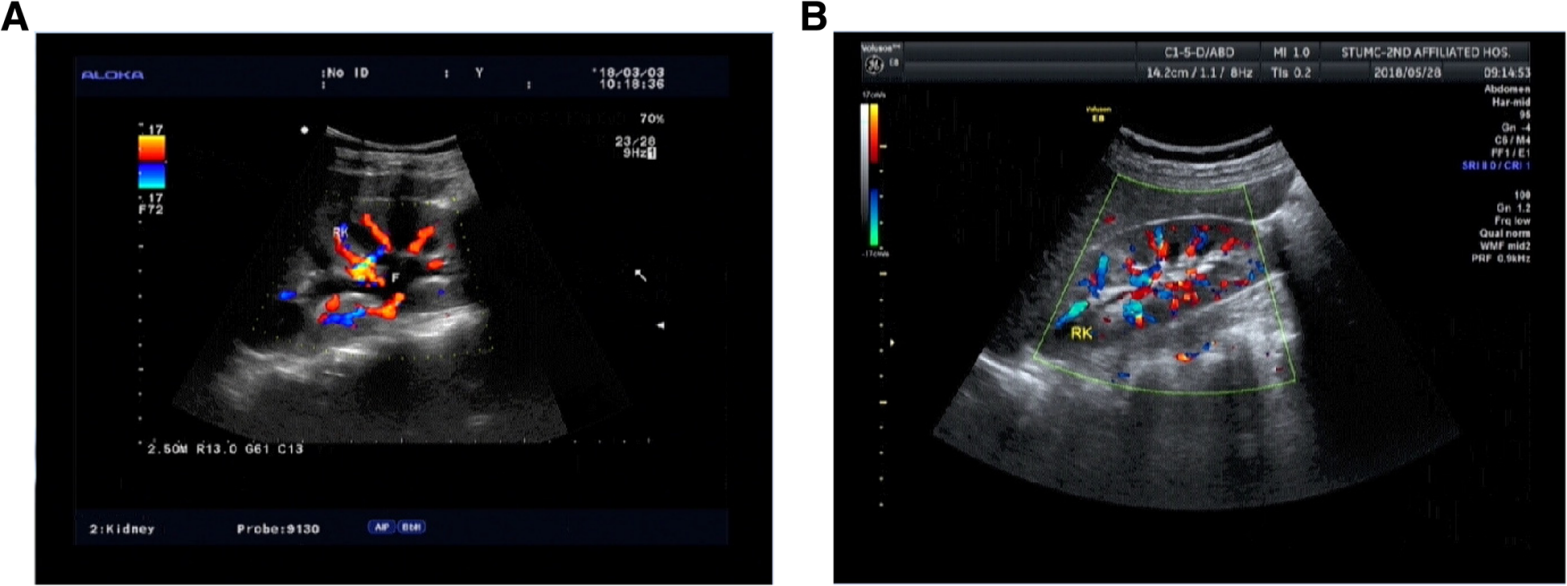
Renal ultrasound indicated: a: Pre-treatment situation: The kidneys have clear outlines, normal shapes, smooth capsules, increased cortical echo, reduced medulla echo, and clear boundaries of the cortex and medulla. b: After treatment: The kidneys have clear contours, normal size and shape, smooth capsules, clear boundaries between the medulla and cortex, and even echoes
